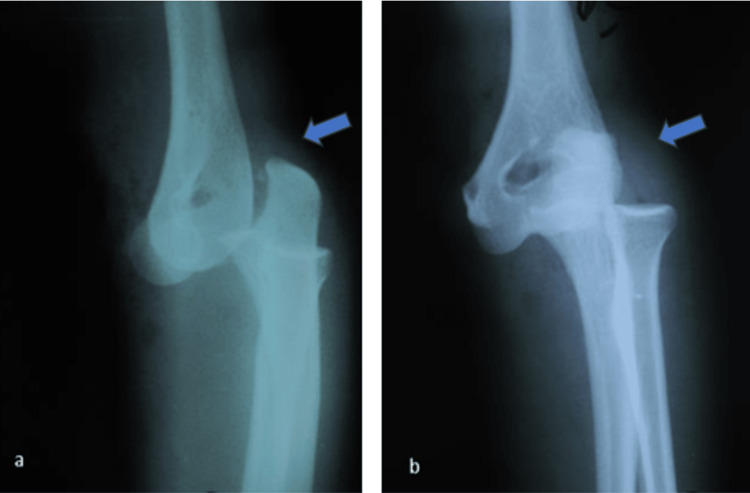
AP (a) and profil (b) radiographs of the elbow showing a posterolateral dislocation (blue arrows).
